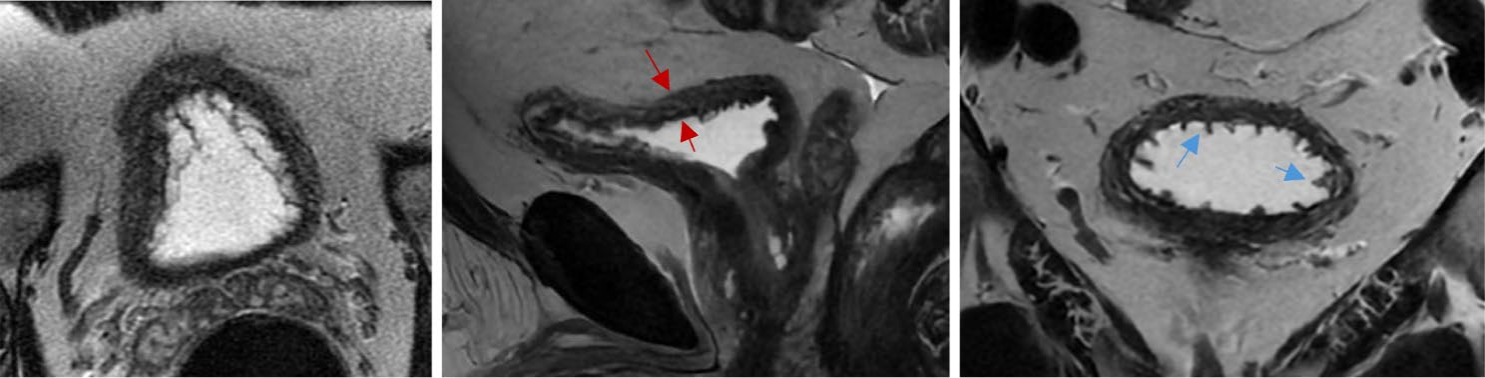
T1/T2 weighted MRI (axial, sagittal, and coronal T2 images) showing circumferential bladder wall thickening (red arrows) and trabeculation secondary to chronic outlet obstruction from benign prostatic hyperplasia (blue arrows)
